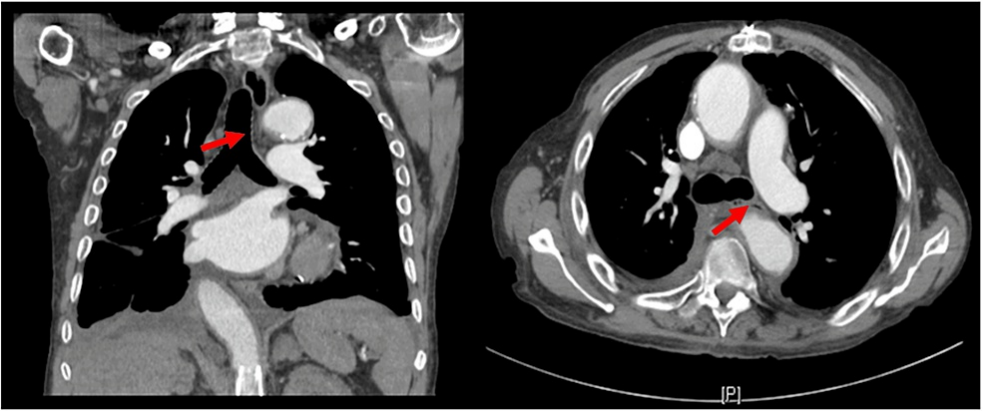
CT chest with and without contrast showed narrowing of the esophagus (see arrow) proximal to the carina due to extrinsic compression between the left main bronchus and the aneurysmal descending thoracic aorta
